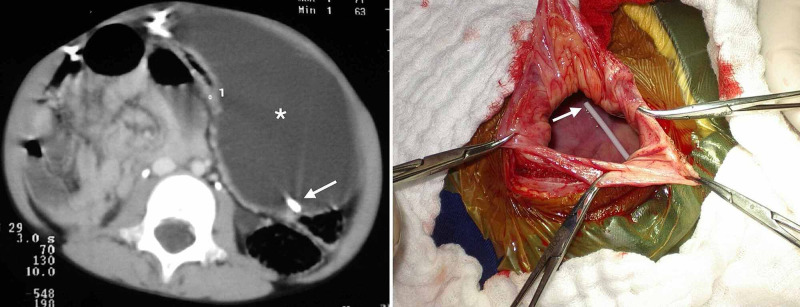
Abdominal pseudocyst. Computed tomography scan of abdomen shows a localized collection of fluid in left peritoneal cavity (*) (left). Intraoperative view of abdominal pseudocyst is shown with dilation of peritoneal cavity, and the distal catheter tip (white arrow) is seen (right).
